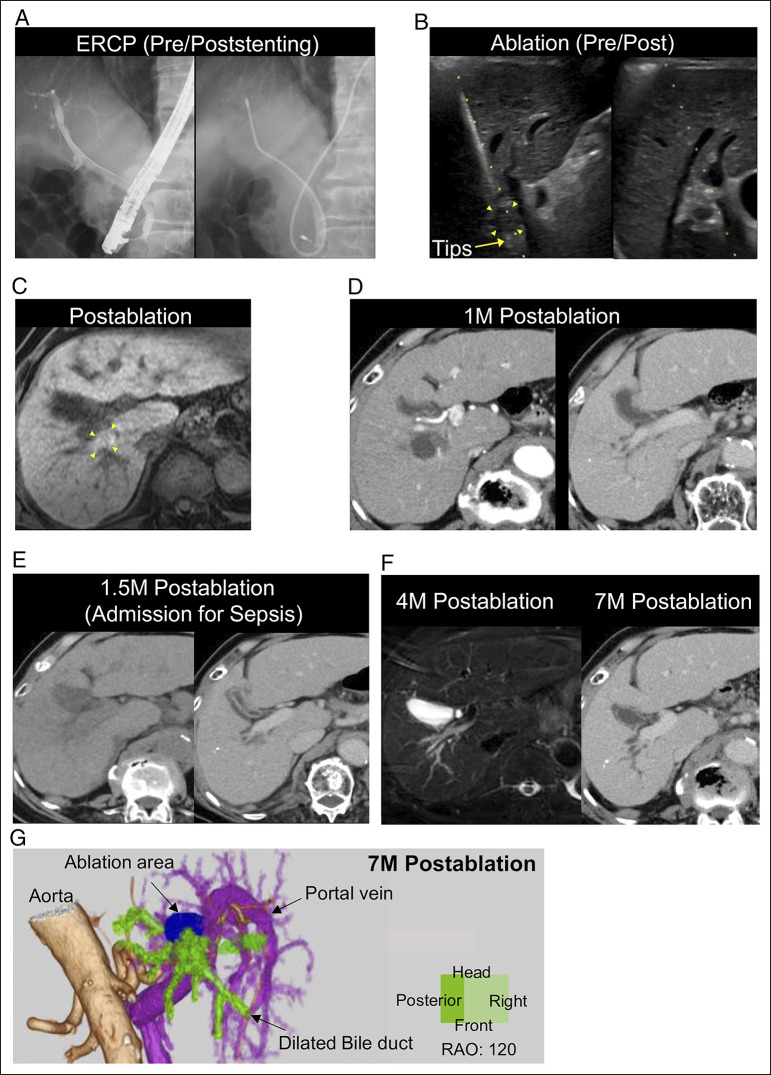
Procedure and imaging course of delayed bile duct injury after hilar HCC ablation. (A) Endoscopic retrograde cholangiopancreatography before and after placement of a 5Fr endoscopic nasobiliary drainage tube into the right anterior segmental duct for intraductal cooling. (B) Ultrasonography obtained during ablation (left) shows the Emprint antenna positioned at the tumor margin (arrowheads), whereas the postablation image (right) confirms an adequate ablation zone without immediate complications. (C) T1-weighted MRI immediately after treatment demonstrates an ablation zone with adequate margin (arrowheads). (D) Contrast-enhanced CT at 1 month shows no local recurrence and no appreciable bile duct dilation. (E) At 1.5 months (≈6 weeks) postablation: left, noncontrast CT at admission for fever; right, contrast-enhanced CT (portal phase) performed during septic shock the following evening. Both show no appreciable biliary dilation. (F) At 4 months postablation, T2-weighted MRI demonstrates definite biliary dilation, which persists at 7 months on contrast-enhanced CT (portal phase). (G) A 3D reconstruction from the 7-month postablation CT (right anterior oblique 120° view) depicts the ablation zone (blue), the biliary tree (yellow-green), portal vein branches (purple), and hepatic arteries (light brown). The biliary narrowing is located at the caudal margin of the ablation zone, anatomically correlating with the progressive ductal dilation. CT, computed tomography; ERCP, endoscopic retrograde cholangiopancreatography; MRI, magnetic resonance imaging.
